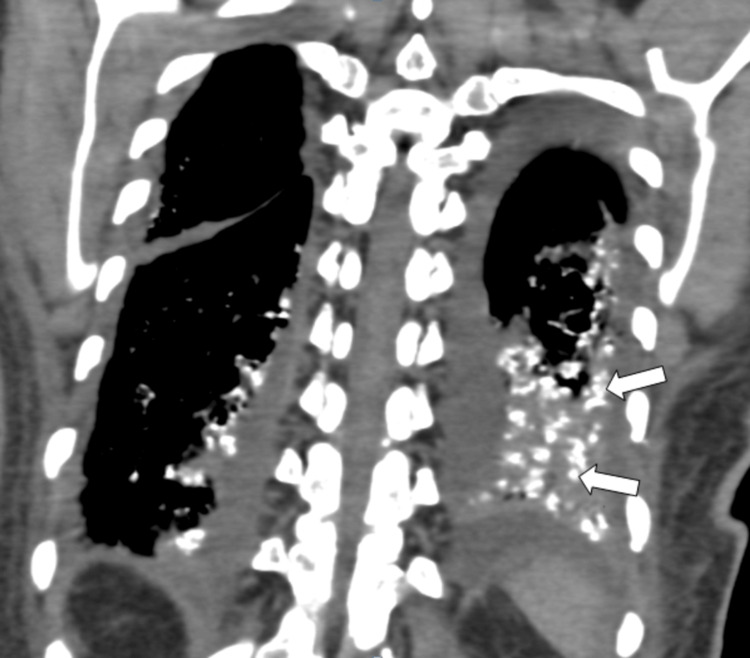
CT coronal image of the same patient showing left lobar peribronchial calcifications (indicated by arrows). CT: Computed tomography
